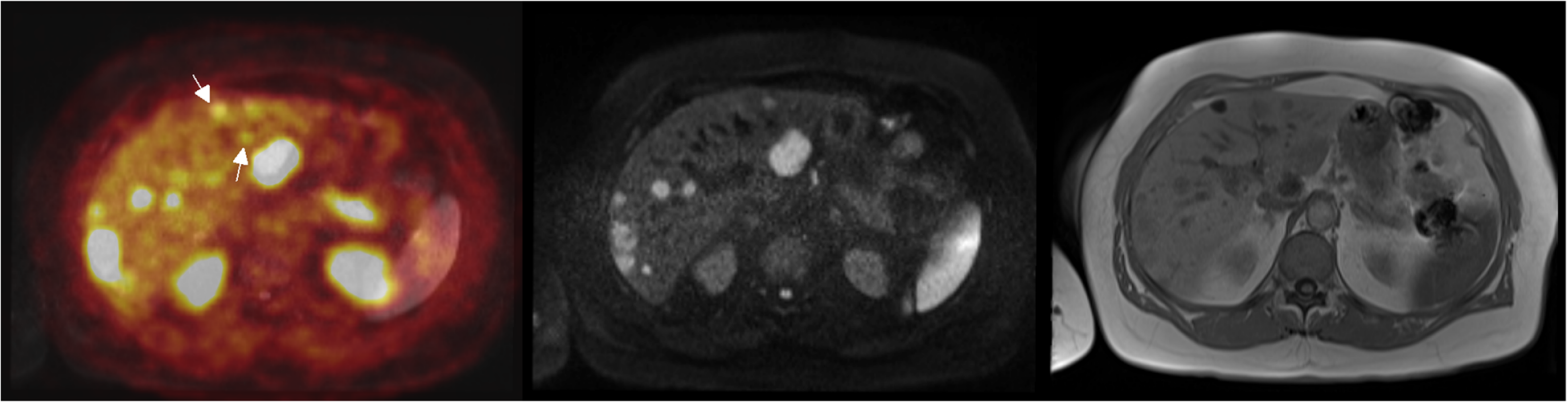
18F-FDOPA PET/MRI of a patient with primary neuroendocrine tumors shows multiple focal uptakes, suggestive of NET liver metastasis. PET and MRI mismatch was seen on the images
